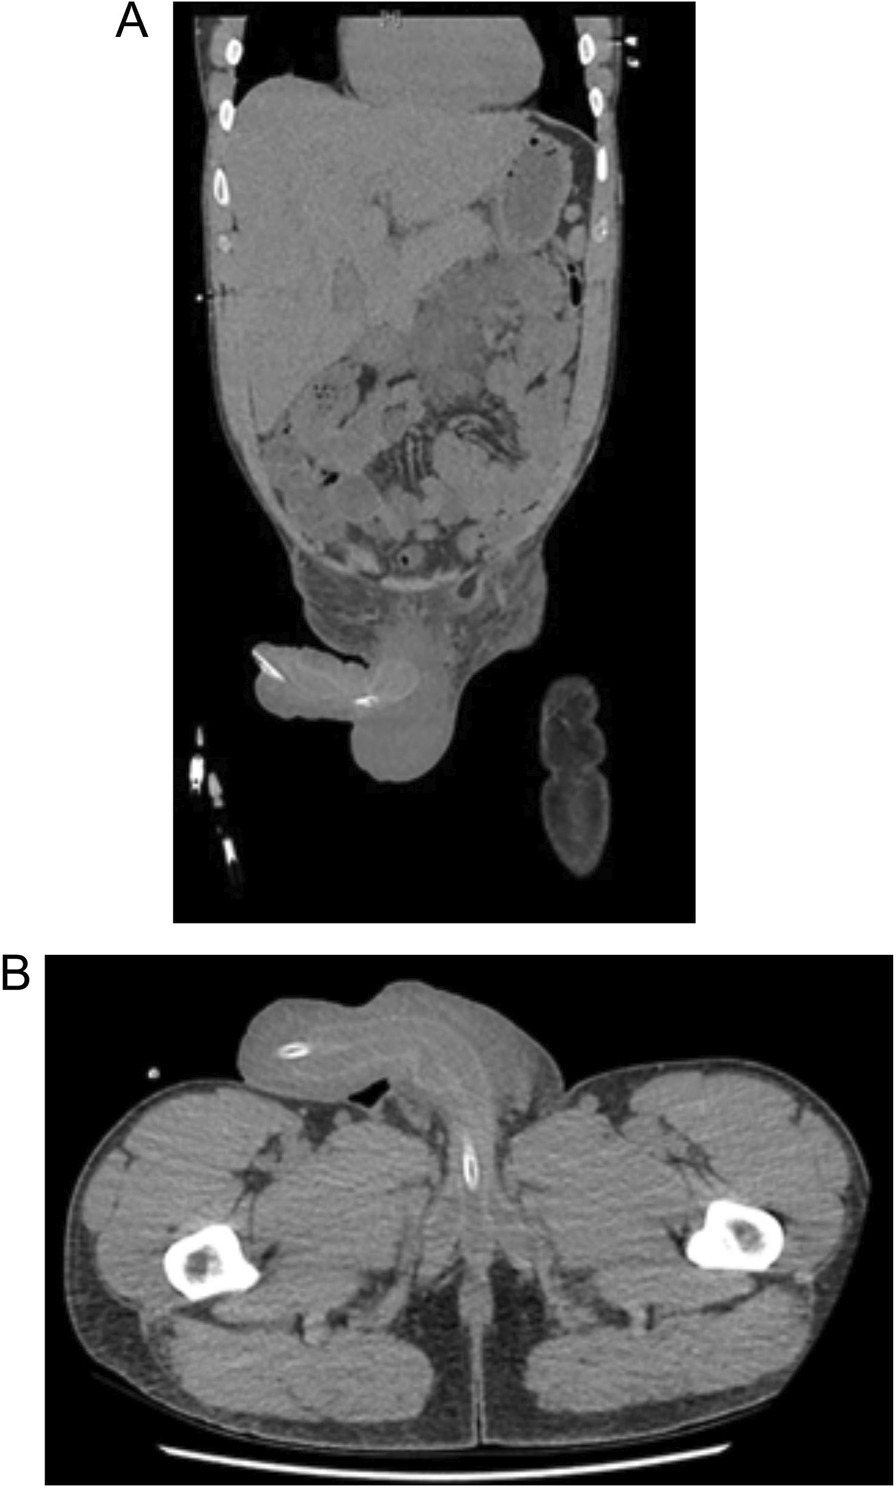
CT scan of the abdomen and pelvis. A Coronal and B Axial sections showing indwelling catheter in situ with extensive penile and pre-pubic soft tissue swelling. No identified abscess or gas locules
